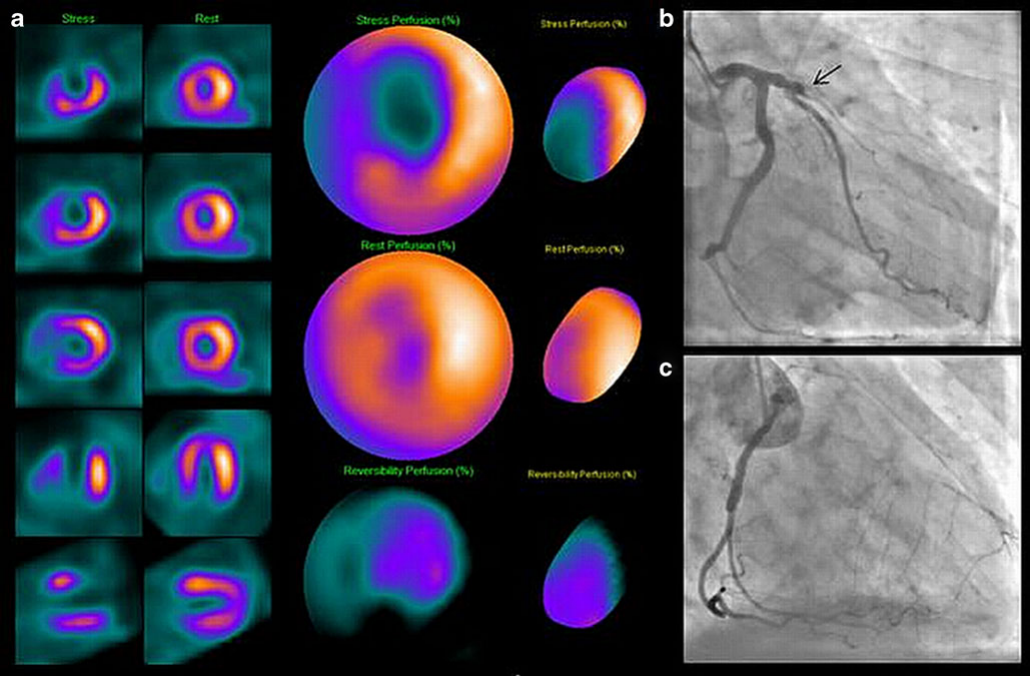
A 71-year-old man with chest pain of suspected cardiac origin. a Regadenoson/rest ^201^Tl MPS. The left ventricular tomograms and the corresponding polar maps show an extensive inducible perfusion abnormality in the left anterior descending coronary artery (LAD) territory. b, c X-ray coronary angiography demonstrates (b) total LAD occlusion (*arrow*) and (c) collateralization from the right coronary artery
